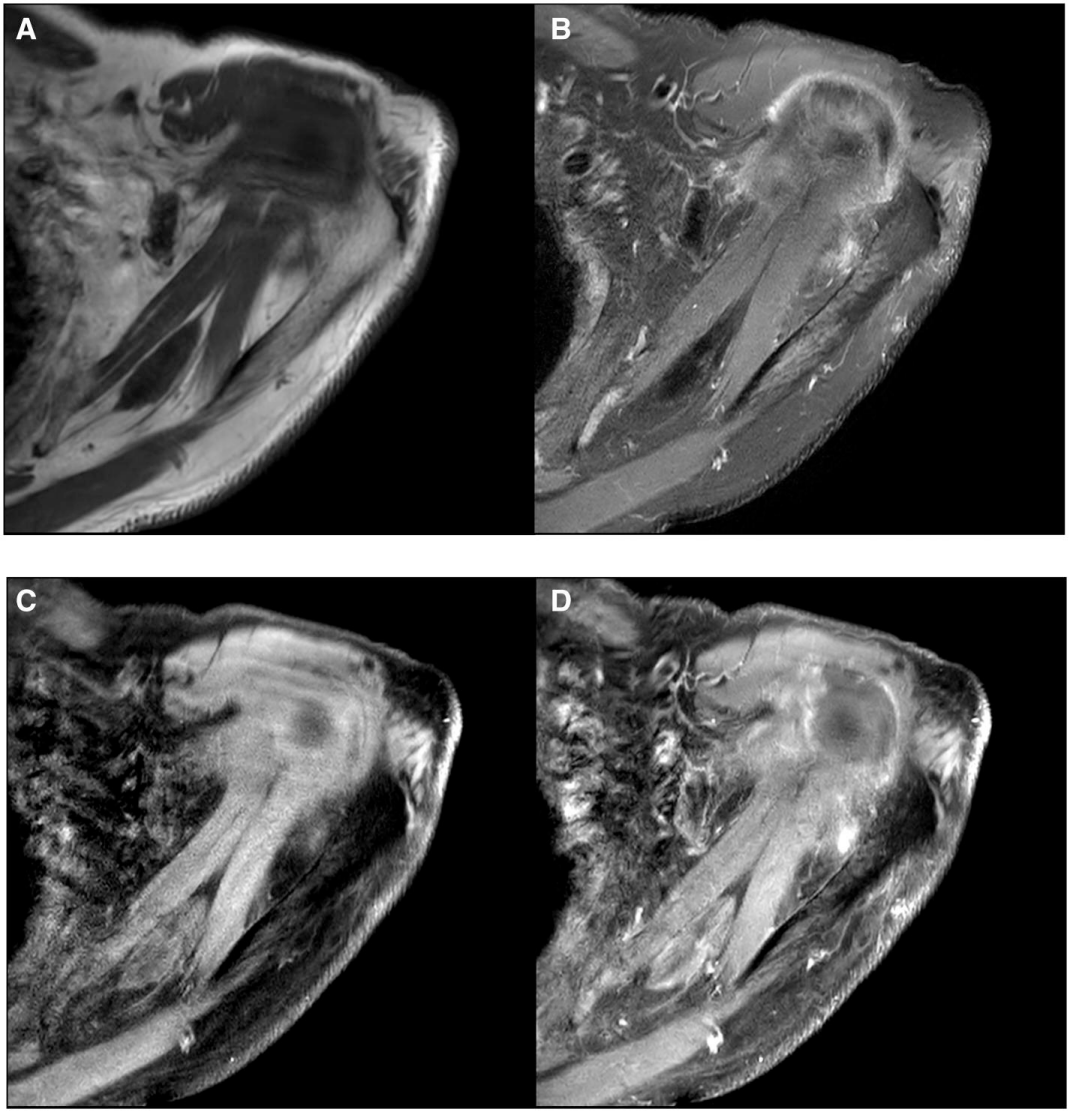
MRI left shoulder. (A) Axial T1: iso to hypointense to muscle. (B) Axial T2 fat-saturated: hypointense to muscle. (C) Axial T1 fat saturated: iso to hypointense to muscle. (D) Axial T1+contrast: enhancement with gadolinium.
